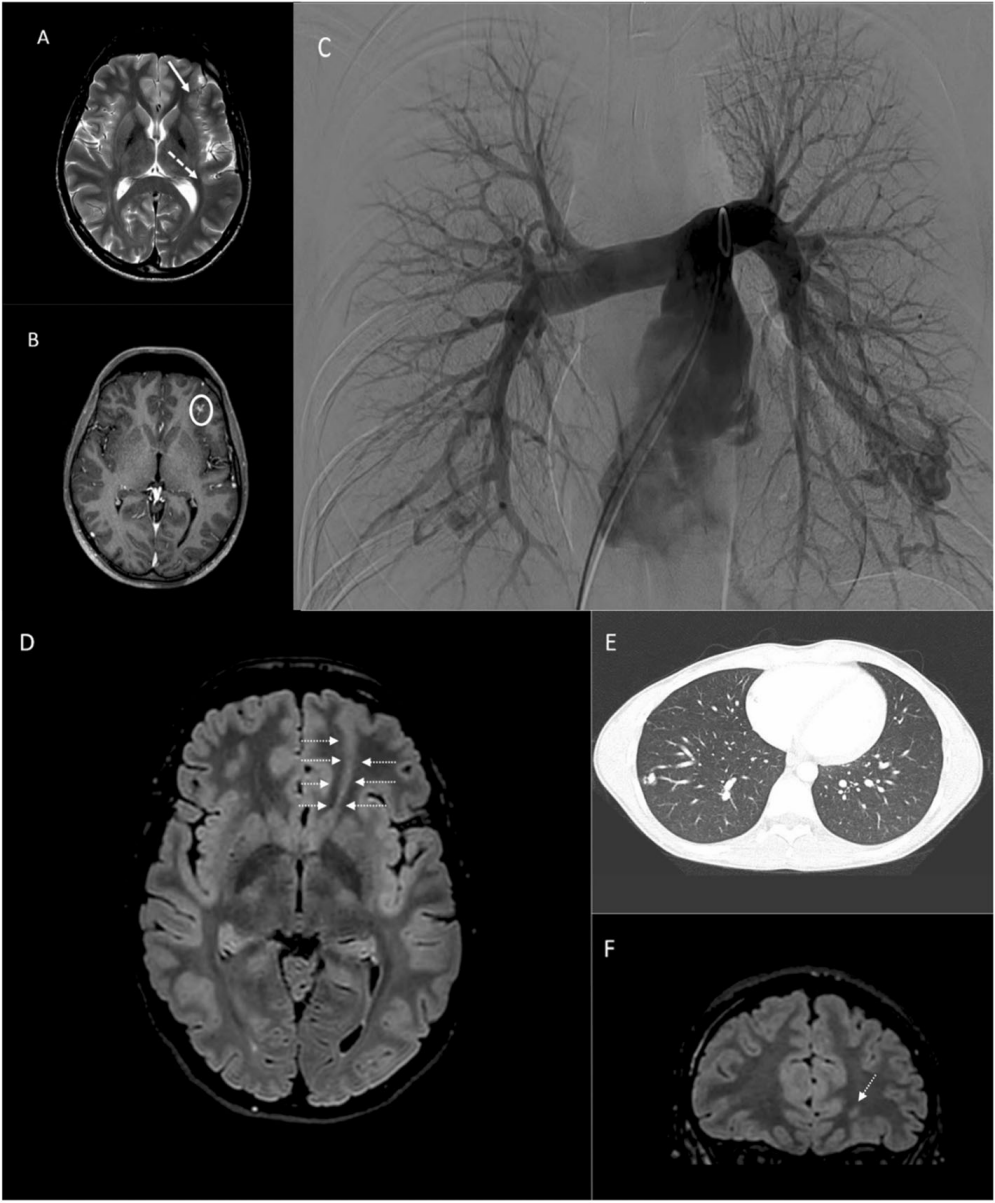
Neuroimaging findings in affected siblings (A-C: Proband, D-F: Probands’s brother). (a-b) MRI images (a: axial Turbo spin echo T2 sequence, b: axial post-gad 3D-T1 images) showing a a cortical development anomaly in left fronto-opercular frontal lobe (white arrow), where the cortex has a polymicrogyria; close to this cortical malformation, the post-gad T1 image b shows a small artero-venous shunt (white circle). In the left, posterior temporal lobe, axial T2 image a show an abnormal thickness of the cortex (white dotted arrow). c Digital angiography of pulmonary arteries shows multiple pulmonary arteriovenous malformations in the left lower lobe. d-f brain MRI Images d: axial Fluid attenuated inversion recovery FLAIR T2 image, f: coronal. FLAIR T2 image) showing a focal blurring in the left frontal lobe, between the medium and superior frontal gyrus, with a transmantle sign (white arrows) reaching the frontal horn of the lateral ventricle, as a cortical focal dysplasia, type II. e Chest CT study shows a pulmonary arteriovenous malformation in the right lower lobe
